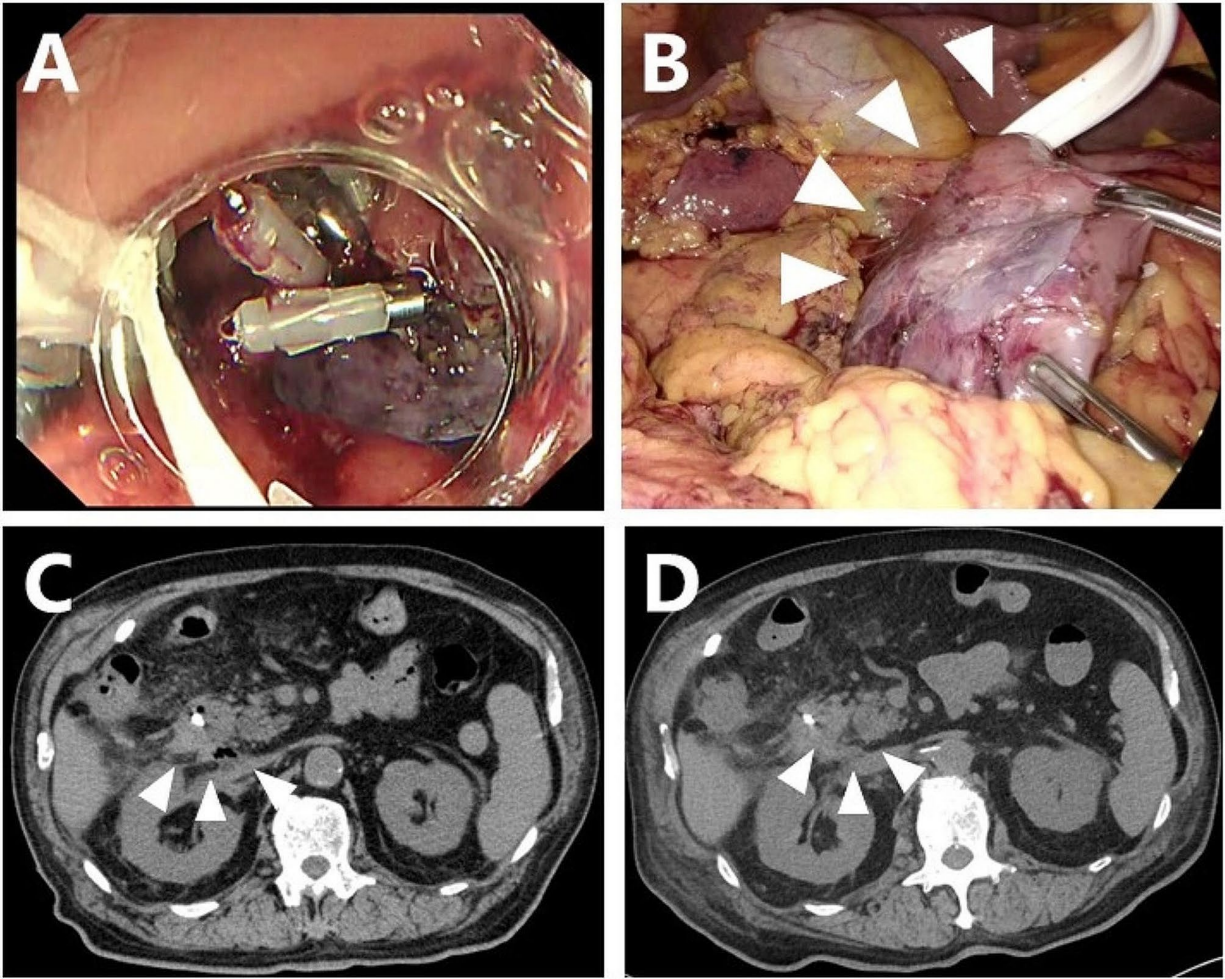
Myoblast sheet transplantation after ESD in case 2. A: The closure of the mucosal defect with clipping was incomplete due to its large diameter. A protruding, thinned ulcerative base after incomplete clipping was seen. B: Two cell sheets were transplanted to fully cover the thinned duodenal wall after duodenal mobilization. C: Abdominal CT three days after transplantation showed a small air bubble at the dorsal side of the duodenum. Neither massive free air nor fluid collection was evident. D: The air bubble on abdominal CT had diminished by seven days after transplantation
